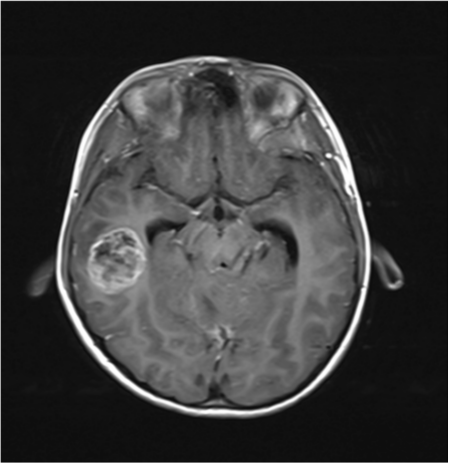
T1-weighted MRI image of the temporal tumor, with obvious contrast
